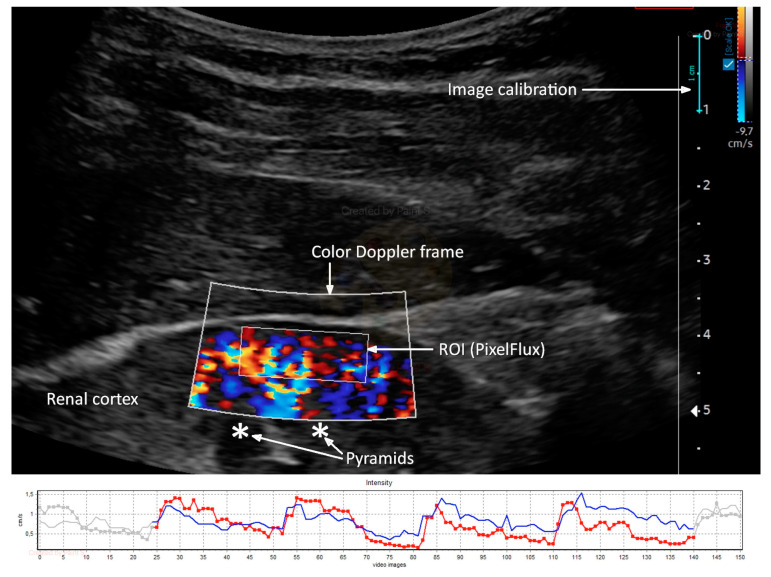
Ultrasound color Doppler image of renal cortex perfusion, analyzed with PixelFlux software (upper part) with a graphical presentation of arterial (red) and venous (blue) flow intensity measurement (lower part). Own materials.
